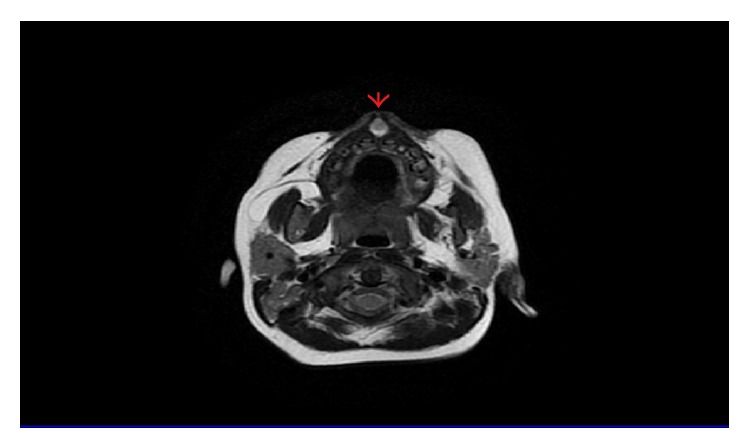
Magnetic resonance imaging shows the lesion that prevented the fusion of orbicularis oris muscles in the midline of the upper lip.
